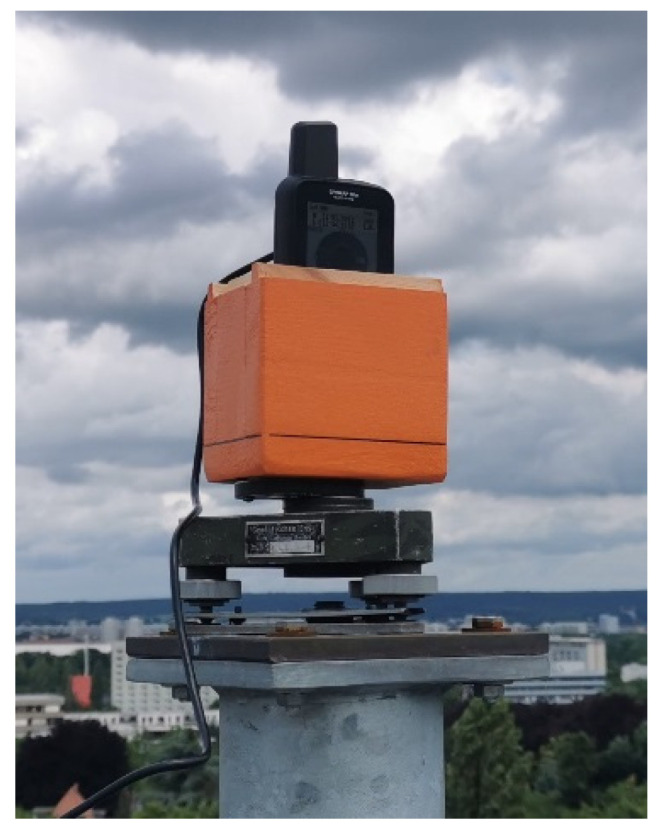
GPSMAP 66sr mounted on tribrach and holder for long-term observations in roof-top environment.
